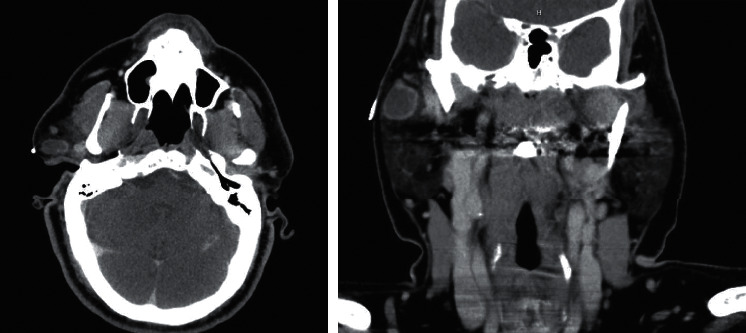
Axial and coronal plane CT neck with contrast demonstrating a 2 cm rim-enhancing lesion within the right parotid gland corresponding to the cestode infection.
